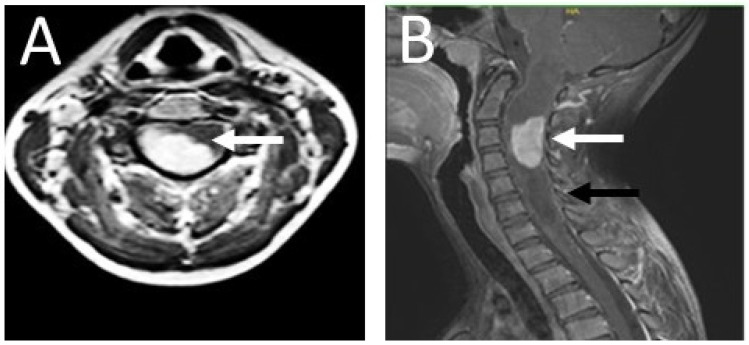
Contrast-enhanced T1 MRI of the patient’s cervical spine. (A) The axial section at the C2 level; (B) the sagittal section. The photos were taken before the procedure. An intramedullary tumor is located in the spinal canal between the C2–C4 levels (white arrow), accompanied by a syringomyelic cavity extending throughout the spinal cord from the hindbrain to the cervical spine at the C7 level (black arrow). Due to the dynamic neurological symptoms, characteristic of the expansive process in the spinal cord, and the need for urgent surgery, imaging diagnostics were limited to the spinal cord and the cervicocranial border. An MRI of the brain was performed at a later stage.
